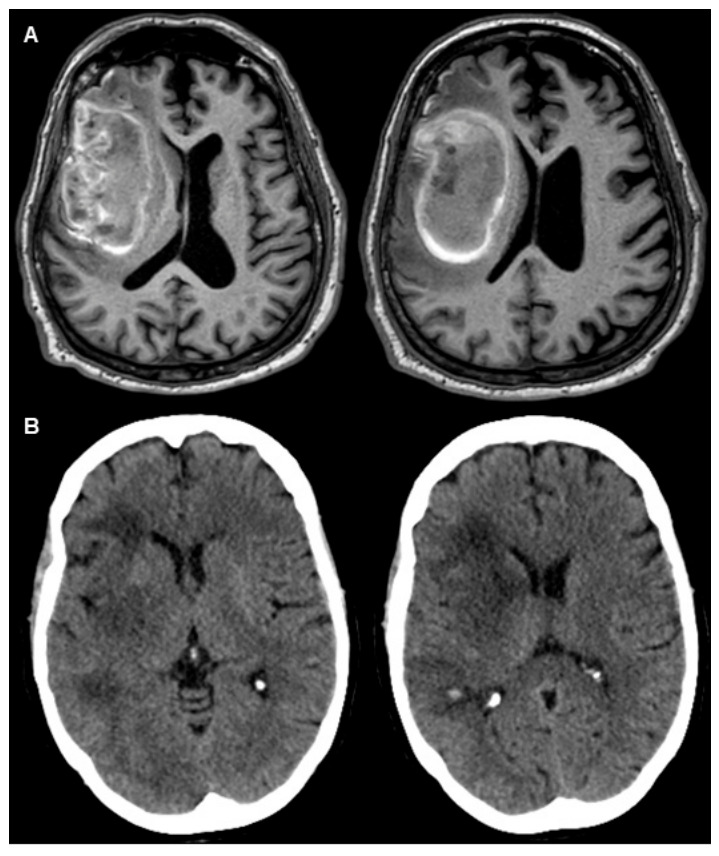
Brain imaging of two patients with unilateral spatial neglect. (A) Axial brain MRI of Patient 1 showing a right-hemisphere hemorrhagic lesion involving fronto-temporo-parietal regions. (B) Axial brain CT of Patient 2 demonstrating a right-hemisphere ischemic lesion involving fronto-insulo-temporal cortico-subcortical regions, with a small focal hemorrhagic transformation.
